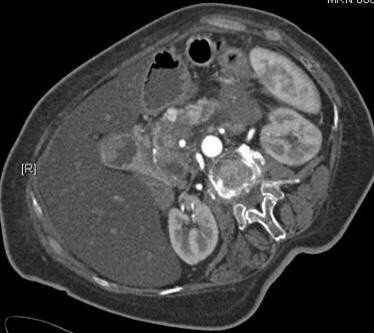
Arterial phase of the computed tomography scan of the abdomen shows a hypervascularized area (arrows) in the pancreatic body.
